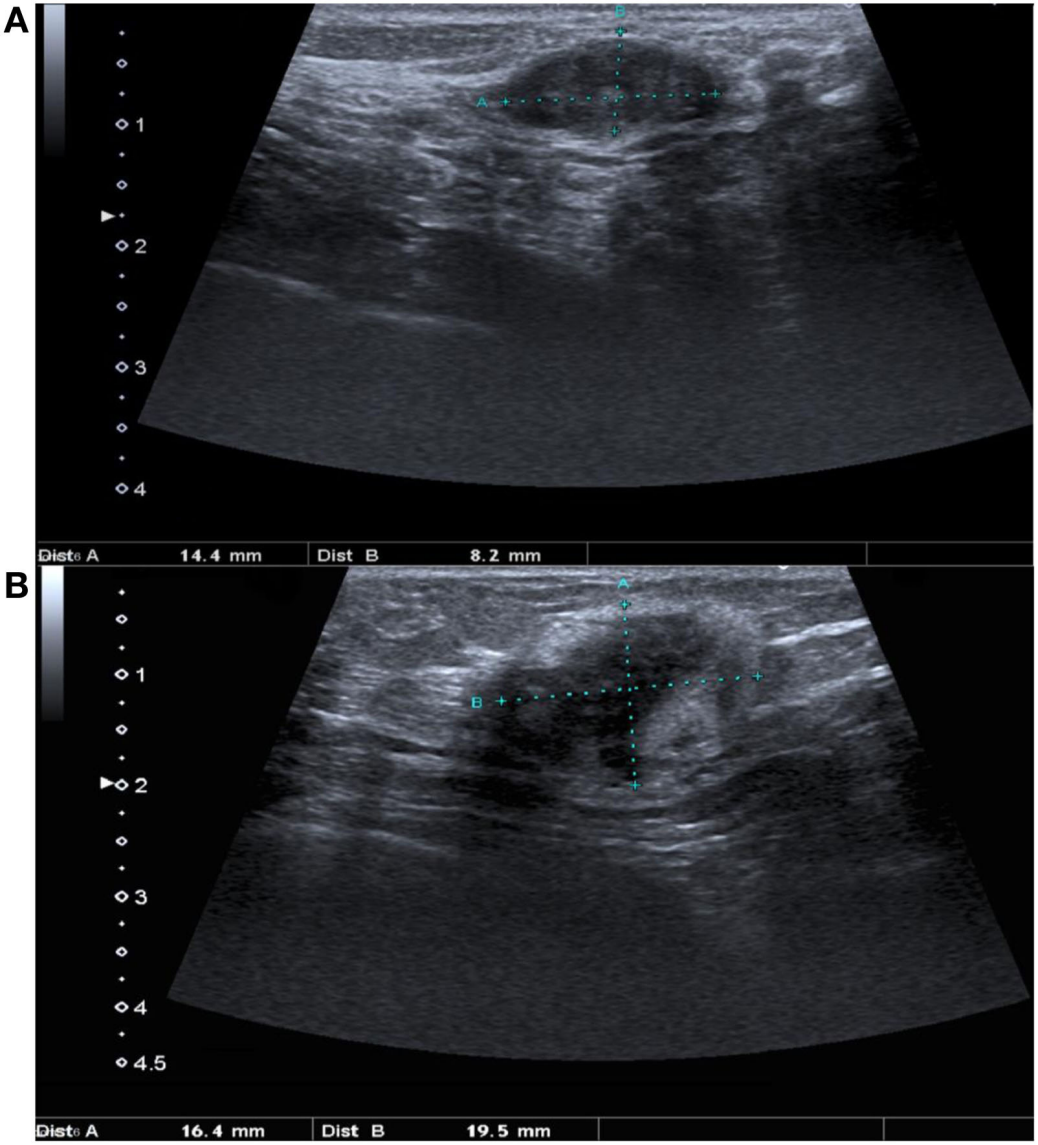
Ultrasound of both breasts demonstrates one of the multiple masses located on the right breast (A) which is characterized by an oval shape, smooth borders and a hypoechoic appearance. The palpable mass located in the upper outer quadrant of the left breast (B) presents an irregular shape and borders with posterior shadowing suggestive of malignancy.
